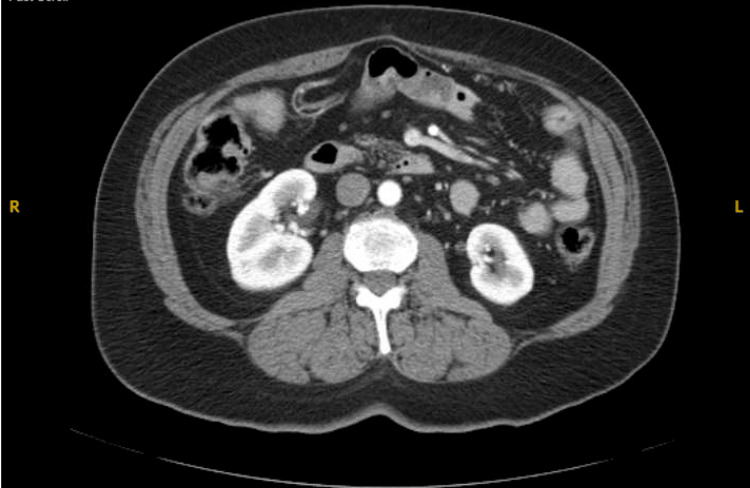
CT scan of chest/abdomen/pelvis CT, computed tomography
